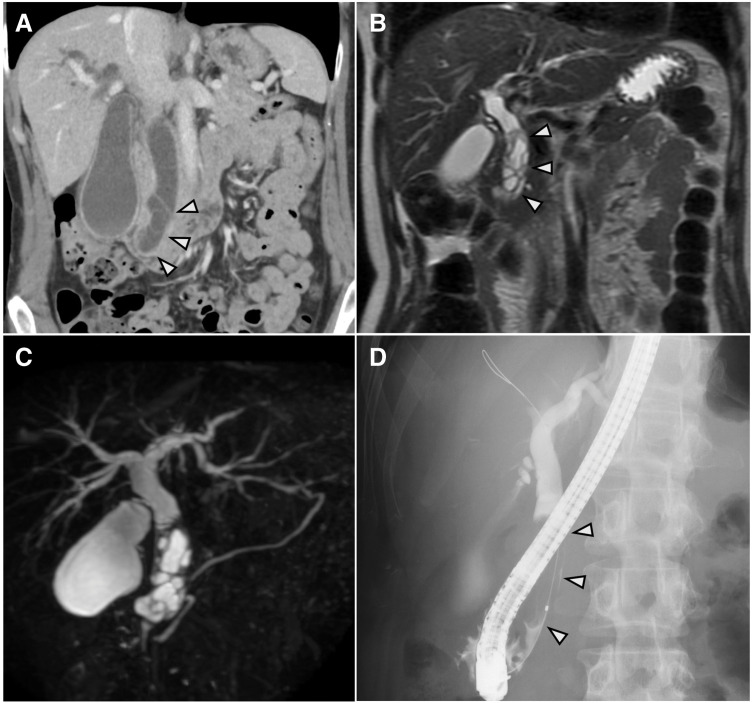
Preoperative imaging findings. (A) Abdominal contrast-enhanced CT (coronal view) during the portal venous phase at the initial visit to the previous hospital: A multilocular cystic lesion is observed in the common bile duct (arrowheads). (B) MRI T2-weighted image (coronal view): A multilocular cystic tumor showing high signal intensity is observed in the common bile duct. The tumor is covered by a thick low-signal capsule, and multiple internal septal structures can be confirmed (arrowheads). (C) MRCP: No anatomical anomalies of the bile duct or pancreaticobiliary maljunction are observed. (D) ERCP: A filling defect is observed in the common bile duct. There is no communication between the tumor and the bile duct (arrowheads). ERCP, endoscopic retrograde cholangiopancreatography; MRCP, magnetic resonance cholangiopancreatography
